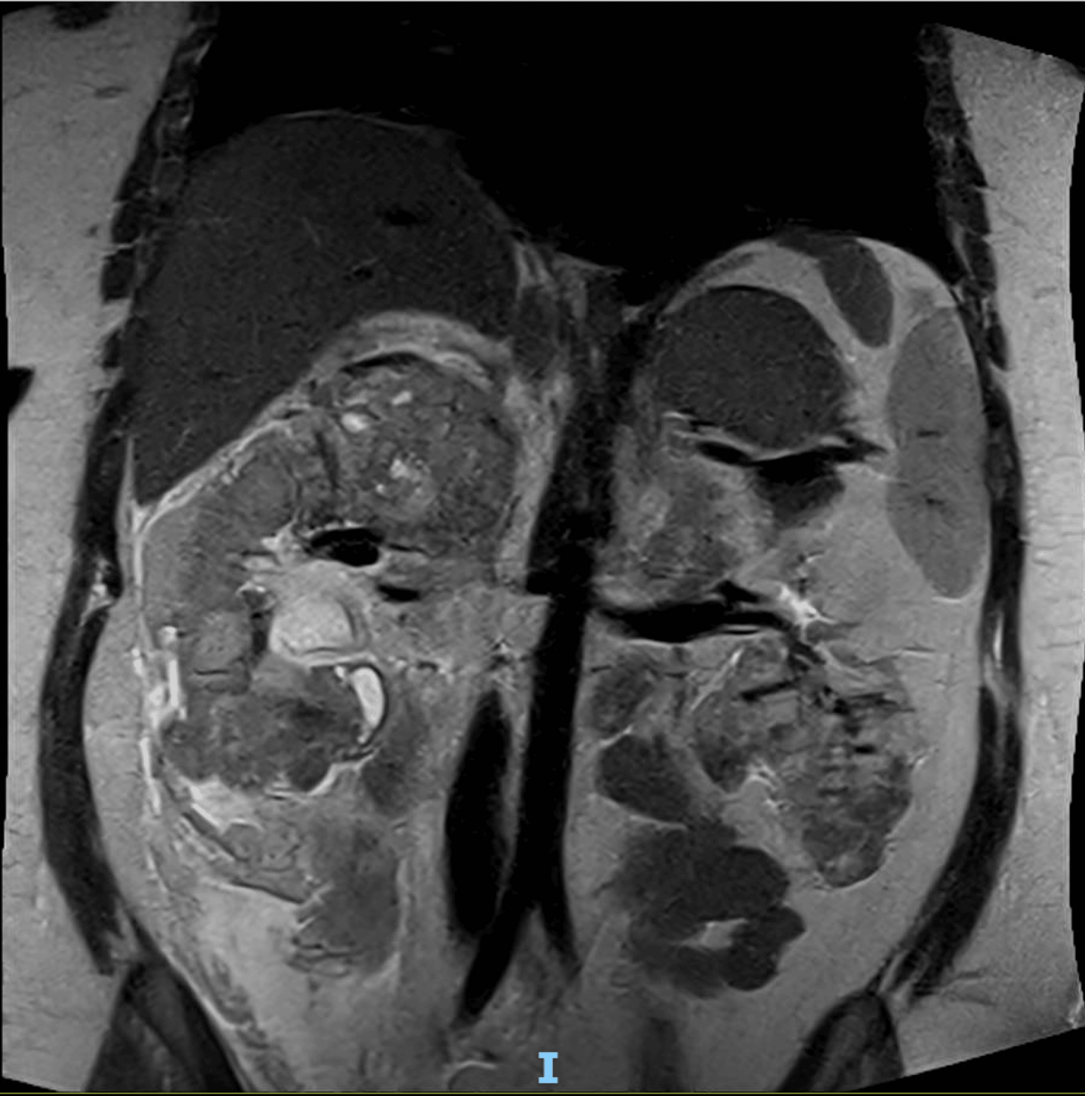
MRI abdomen T2-weighted: coronal thin slice The MRI shows grossly distorted kidneys and innumerable bilateral intrarenal angiomyolipomas.
